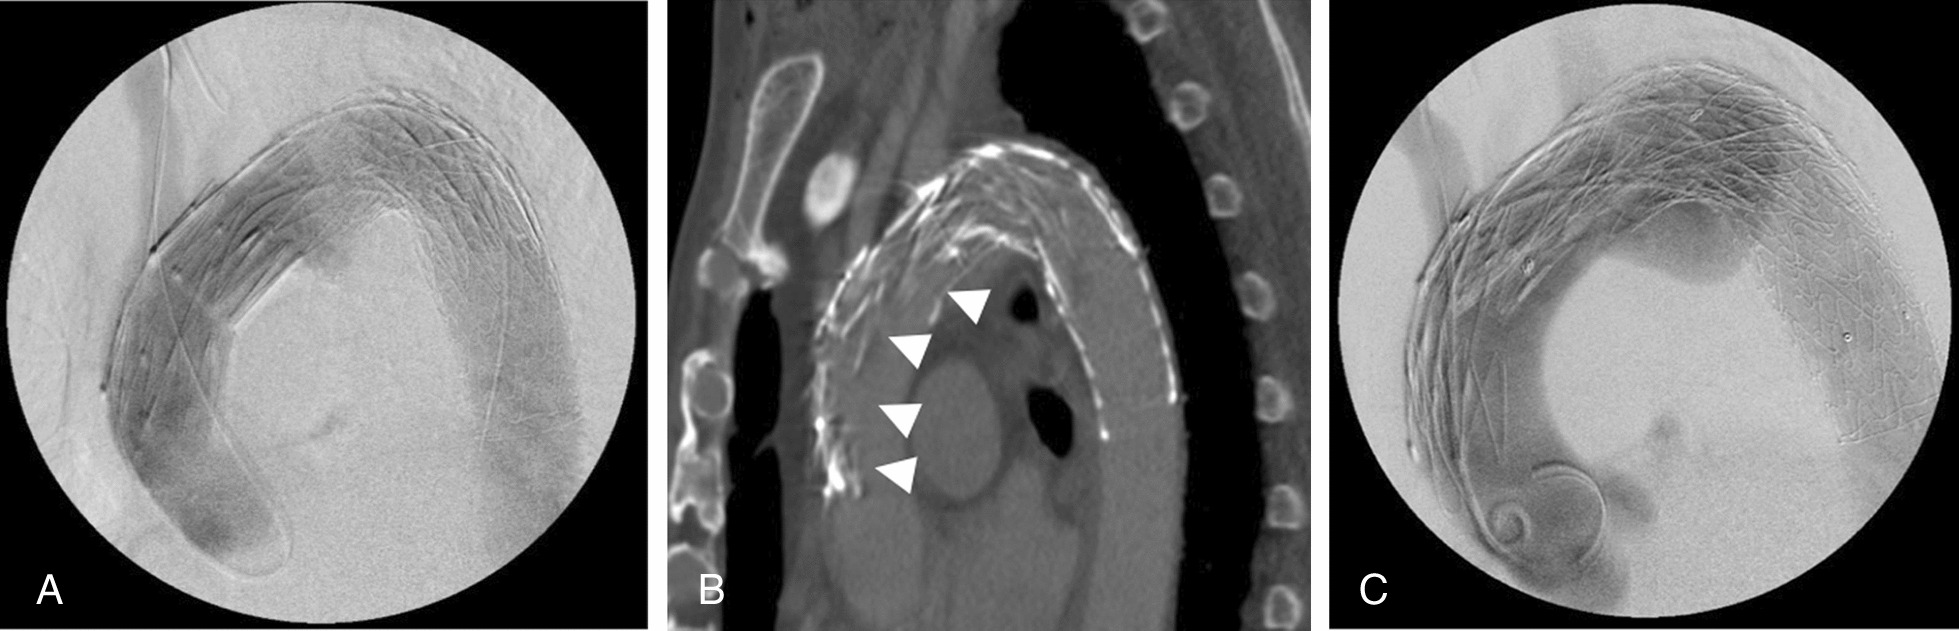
A Final angiography revealed preservation of the brachiocephalic artery and debranching bypass flow and demonstrated full exclusion of the aneurysm. B Computed tomography shows proximal collapse of the endograft (arrow heads). C At additional TEVAR, angiogram showed a type I an endoleak and proximal collapse of the stent graft
